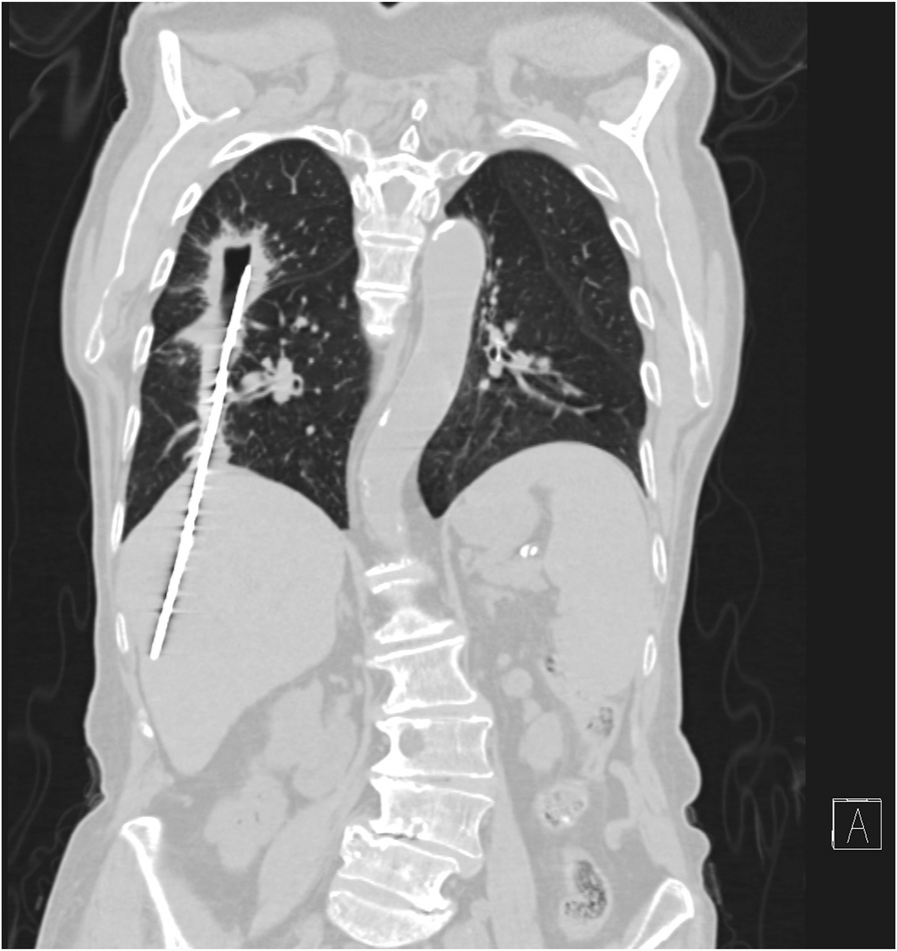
Coronal slice of thoraco-abdominal CT. There is an 18 cm metallic foreign body extending from right liver lobe (segment V and VIII), transdiaphragmatic, to the right upper lobe
